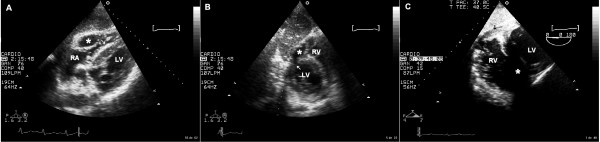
Panel A: transthoracic echocardiography, modified four chamber subscostal view showing the dissecting tract through right ventricular free wall forming an intramural neo-cavity (asterisk), the arrow indicates the re-entry point of dissecting tract into right ventricle chamber. Panel B: transthoracic echocardiography, modified short axis subcostal view showing rupture of the inferobasal septum at the point that originates the right ventricular wall dissection (arrow), asterisk indicates the dissected neo-cavity. Panel C: postoperative transesophageal echocardiography, transgastric short axis view showing thickened basal segments of the right ventricle and inferobasal septum without visible dissecting tract (black arrow), note increased echoreflectivity at this site. Acoustic shadow caused by prosthetic patch has been indicated (asterisk). Abbreviations: LV, left ventricle, RA, right atrium, RV, right ventricle.
